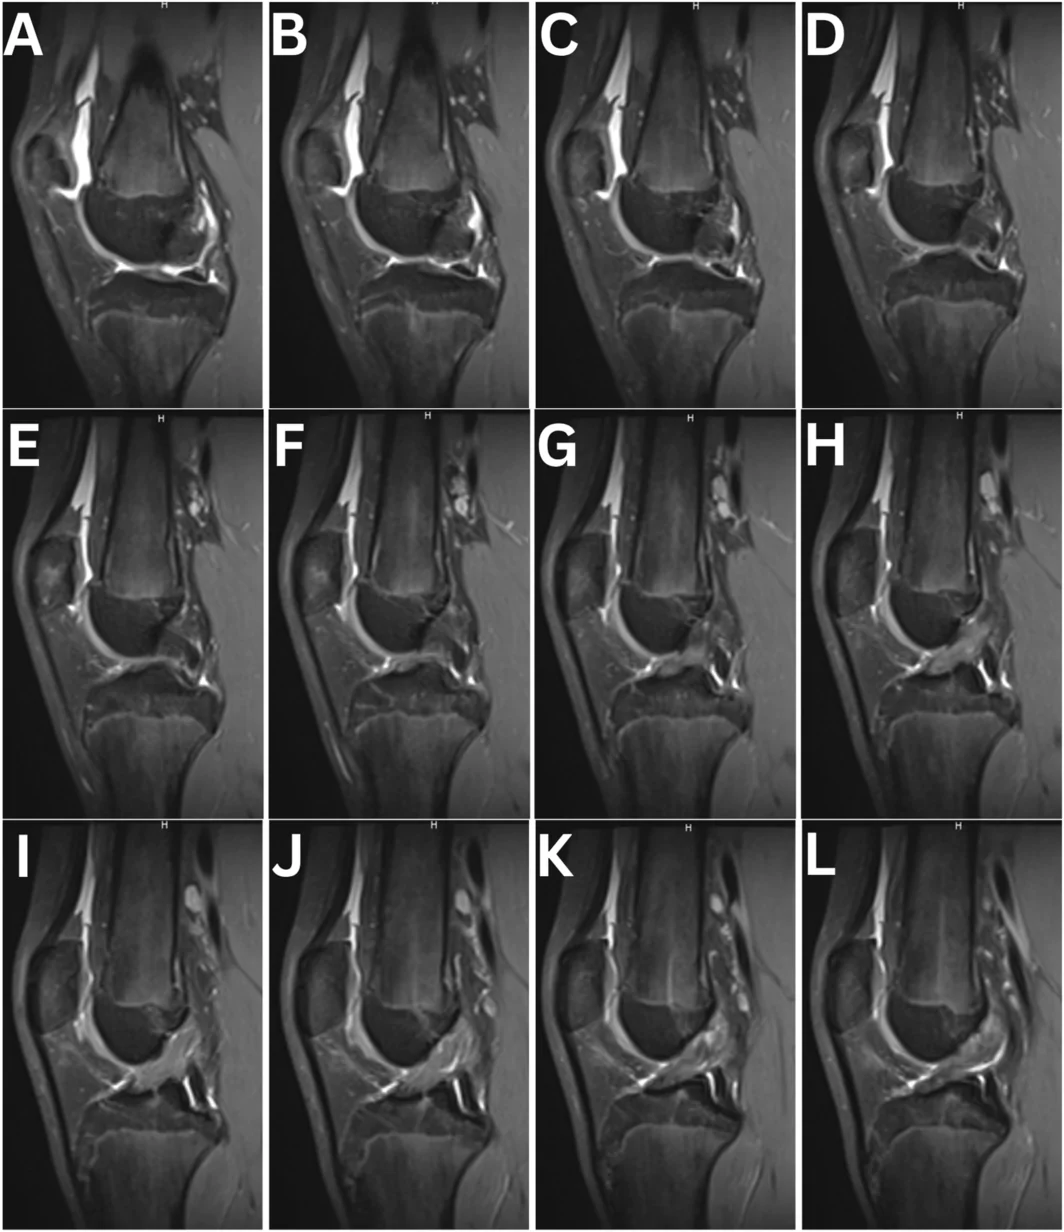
Initial sagittal MRI. Consecutive sagittal fat‐suppressed proton density–weighted images of the right knee (A–L, medial to lateral) show a high‐grade ACL tear with features of complete rupture, including loss of normal fiber continuity, fluid in the intercondylar notch, and a thickened, wavy, horizontalized remnant with increased intrasubstance signal. Joint effusion is present, while the PCL is intact. Overall, the findings are most consistent with complete ACL rupture, although a high‐grade partial tear cannot be excluded.
